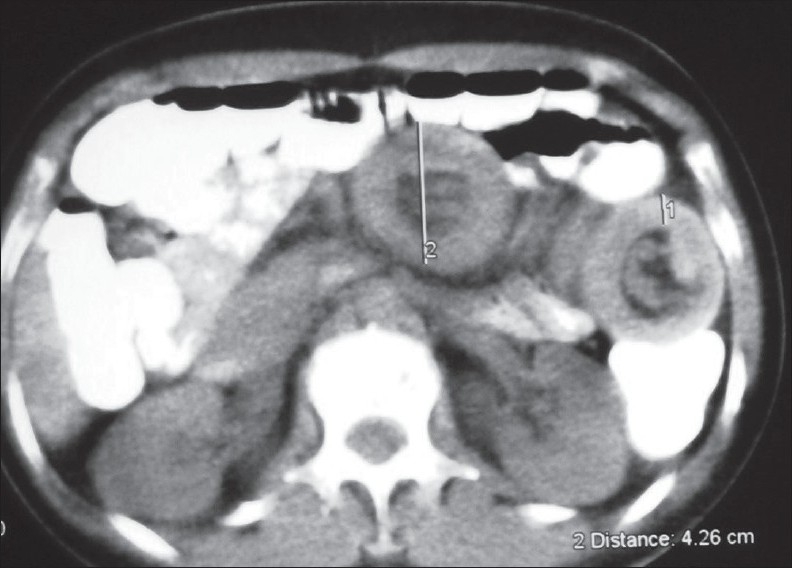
A contrast-enhanced abdominal CT scan with oral contrast administration demonstrated classic bowel-in-bowel appearance of small intestinal intussusception
